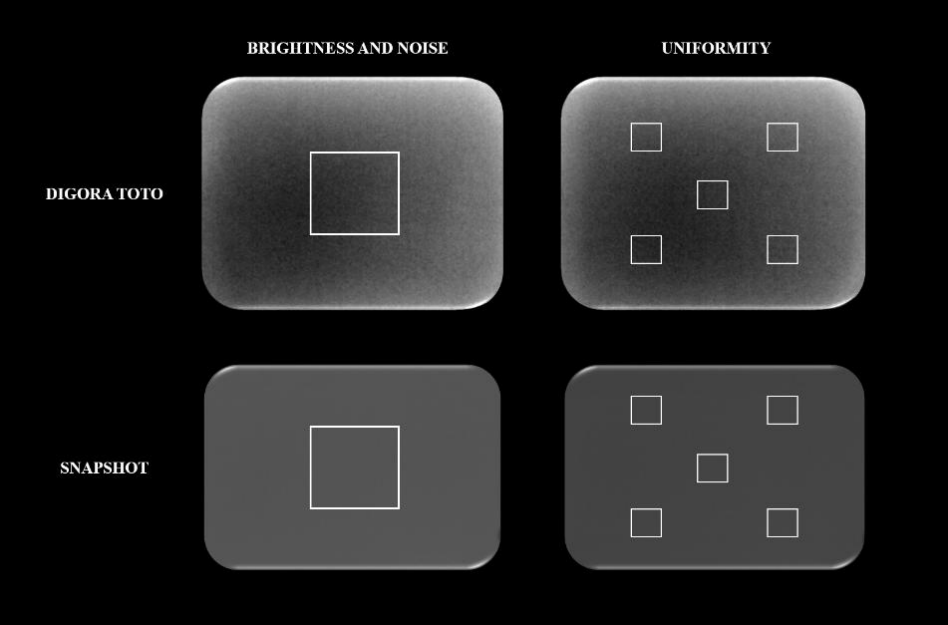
Radiographs with empty white squares representing the ROIs for brightness, noise, and uniformity evaluation. *ROI, region of interest*
